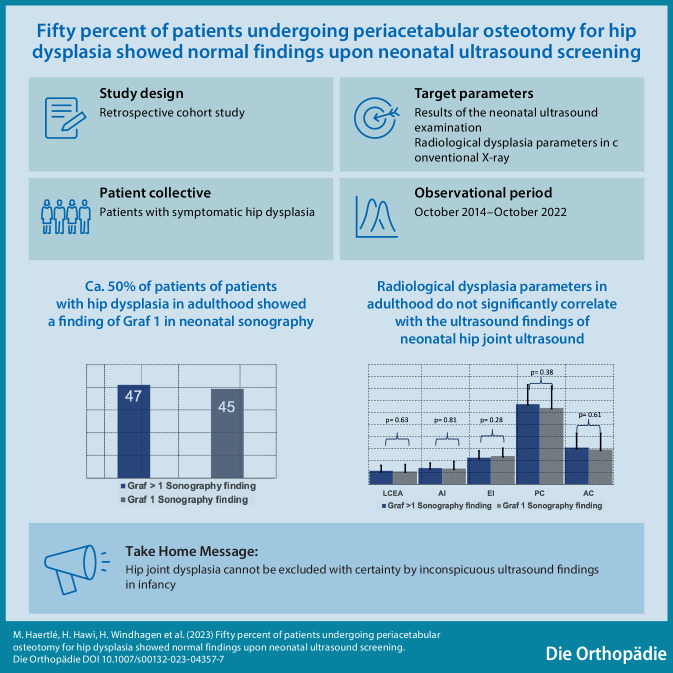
A universal nationwide ultrasound screening program was introduced in Germany in 1996 to reduce the incidence of developmental dysplasia of the hip (DDH). Despite the introduction of this program, a rise in young adults with symptomatic acetabular undercoverage seeking treatment in hip preservation centers is being seen. This study shows that acetabular undercoverage cannot be ruled out based on a normal ultrasound finding at neonatal screening.
